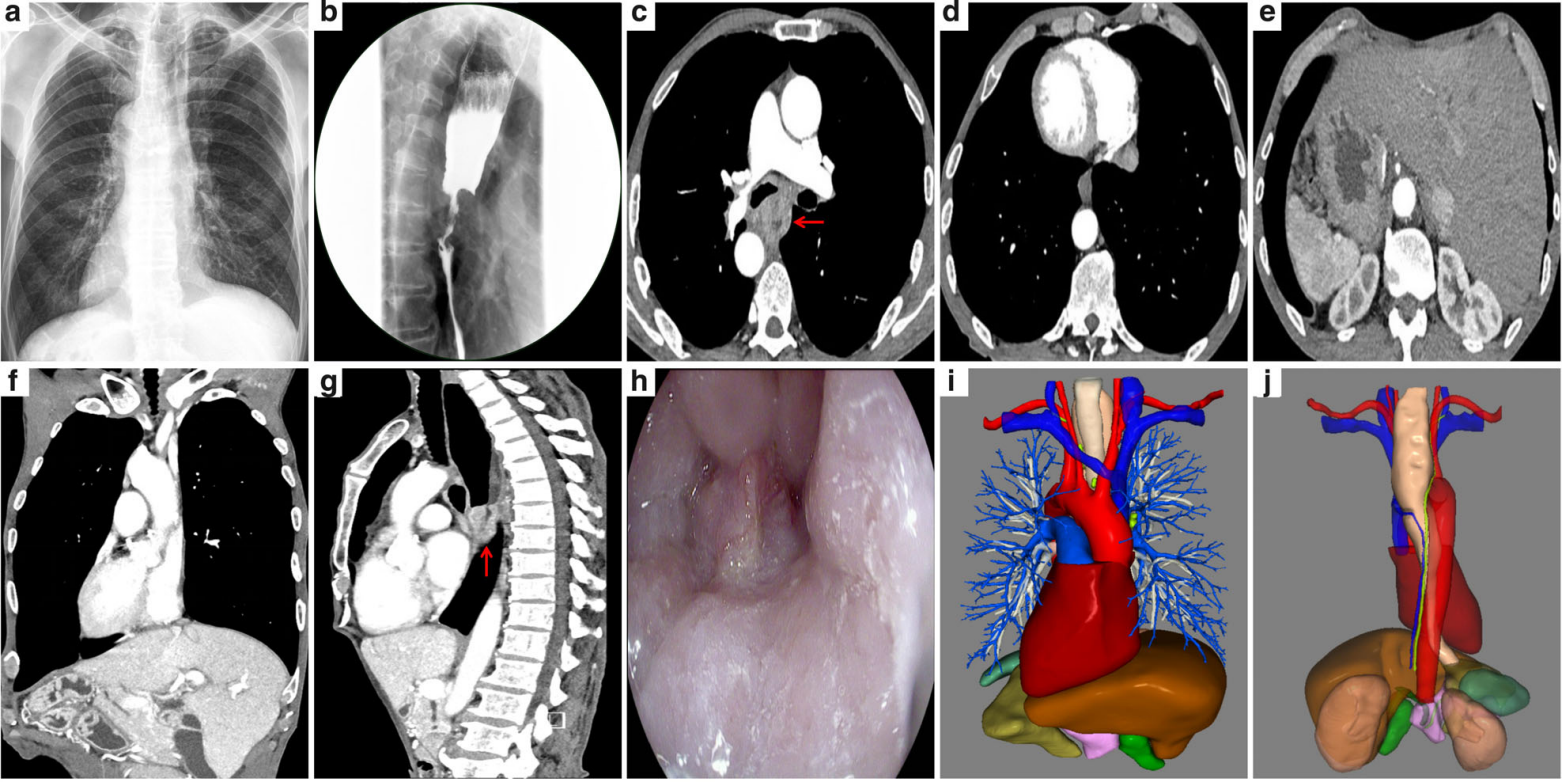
Preoperative imaging examination confirmed the presence of SIT and middle third esophageal cancer. (a) Chest X‐ray showing dextrocardia and a right aortic arch; (b) Barium swallow showing the tumor in the middle third esophagus; Cross section (c) and sagittal section (g) of CT showing the tumor (arrow); Chest CT (d), Abdominal CT (e) and coronal section of CT (f) showing SIT; (h) Endoscopic ultrasound revealed an ulcerated esophageal tumor; Anterior (i) and posterior views (j) of 3‐D images demonstrating the surrounding structures around the esophagus.
